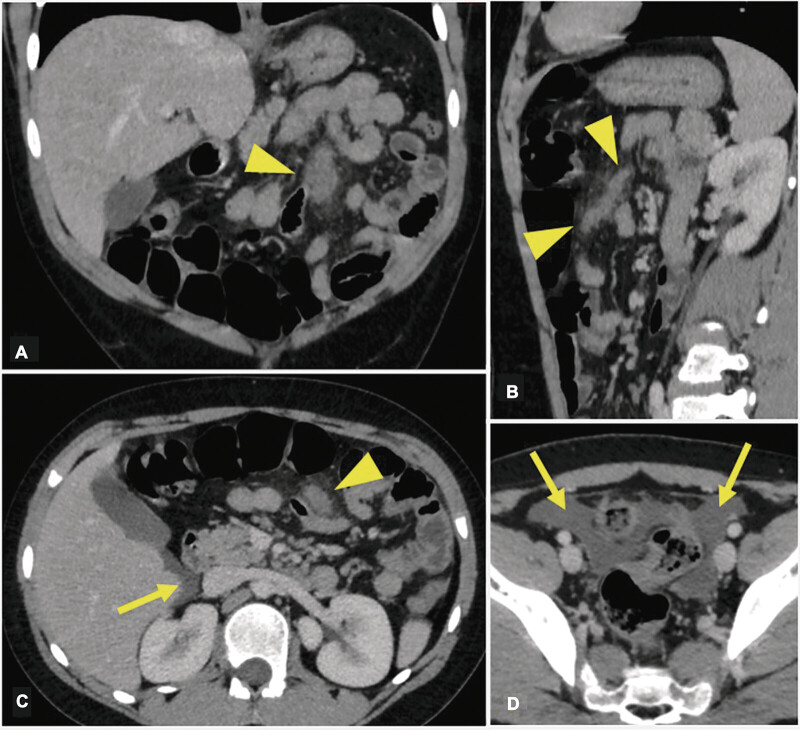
Contrast enhanced CT scan of the abdomen performed on December 25, 2022, shows an ill-defined oblong dense mesenteric root structure (arrowhead) in the left para-sagittal aspect of the upper abdomen, on the coronal (
